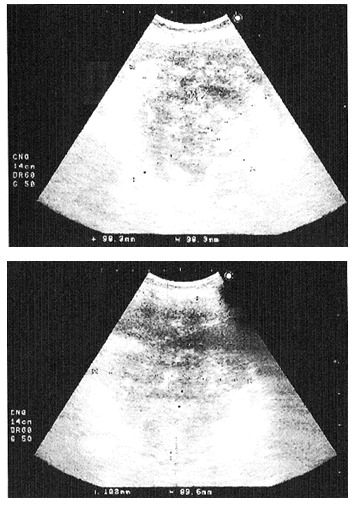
Ultrasound aspects of the retroperitoneal metastasis root of tooth 36
